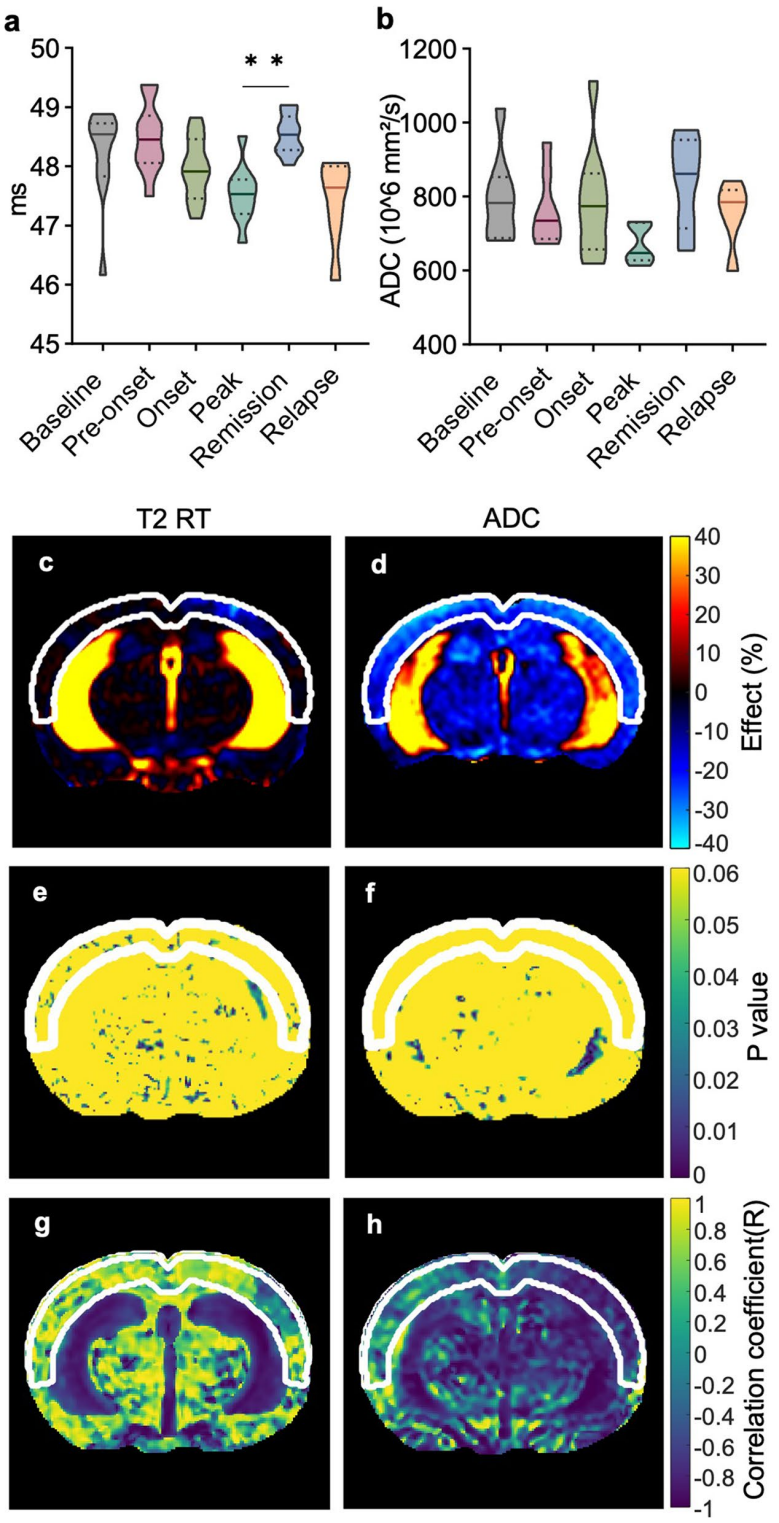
Detection of disseminated water biophysical properties by quantitative MRI. a Longitudinal variations in cortical T2 RT in ms (*n* = 9 onset; *n* = 8 baseline, pre-onset; *n* = 7 peak; *n* = 6 remission *n* = 5 relapse); b ADC in × 10^6^ mm^2^/s (*n* = 12 baseline, pre-onset, peak; *n* = 11 remission; *n* = 6 relapse). *P* < 0.01. c, d Effect maps of percentage changes in T2 RT and ADC from peak to baseline reveal a focal reduction in T2 RT and a disseminated reduction in ADC in the cortex. e–h A relapsing–remitting paradigm applied to T2 RT and ADC maps demonstrates a lack of specificity for cortical alterations
